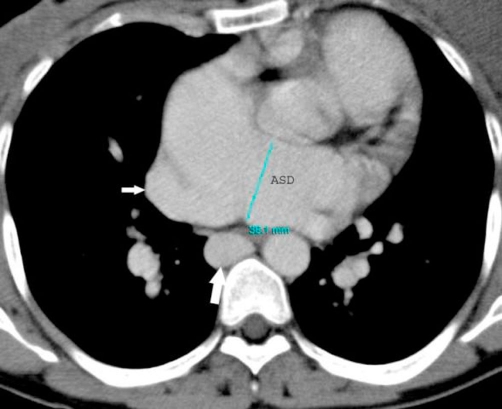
Contrast-enhanced axial CT image demonstrates enlarged azygos (white large arrow), abnormal return of right-sided pulmonary veins into the right atrium (white small arrow) and atrial septal defect (ASD).
